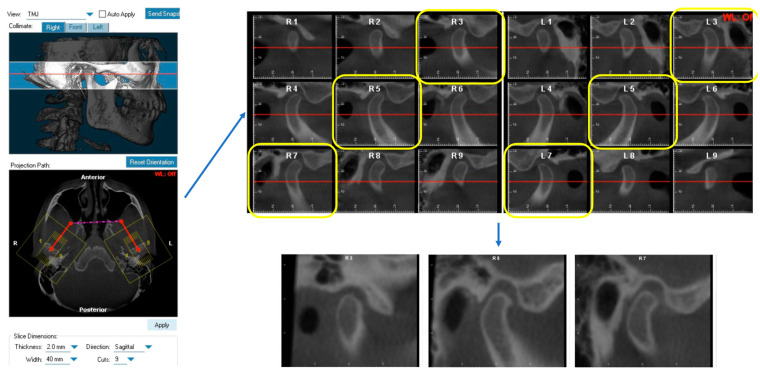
Generation of sagittal TMJ slice cuts from CBCT.
